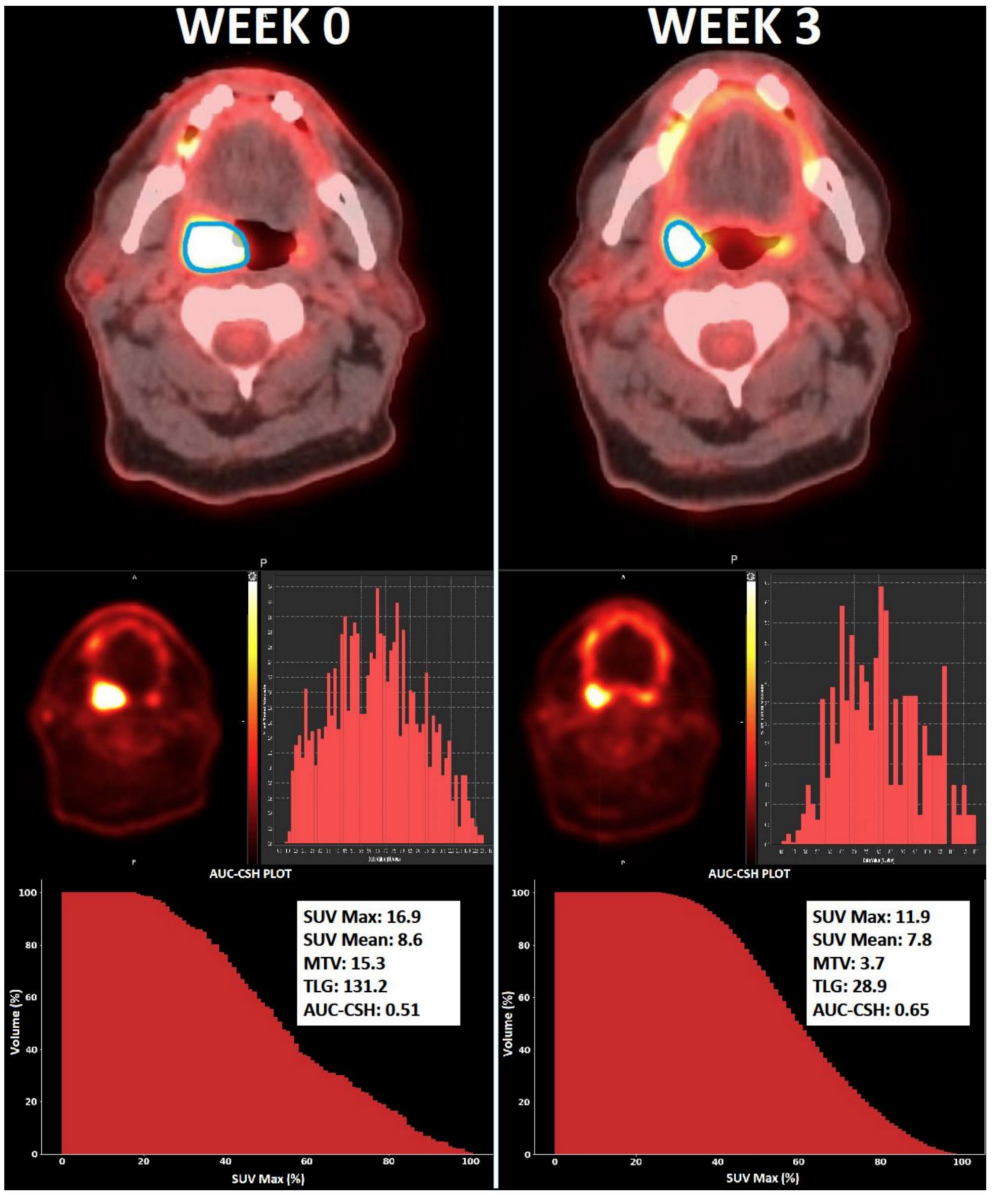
Case example of a patient with stage IVa HPV-positive tonsil squamous cell carcinoma (T2N1M0) who exhibited a favourable mid-treatment FDG-PET imaging response after chemo-radiotherapy and remained disease-free 5 years post-diagnosis
